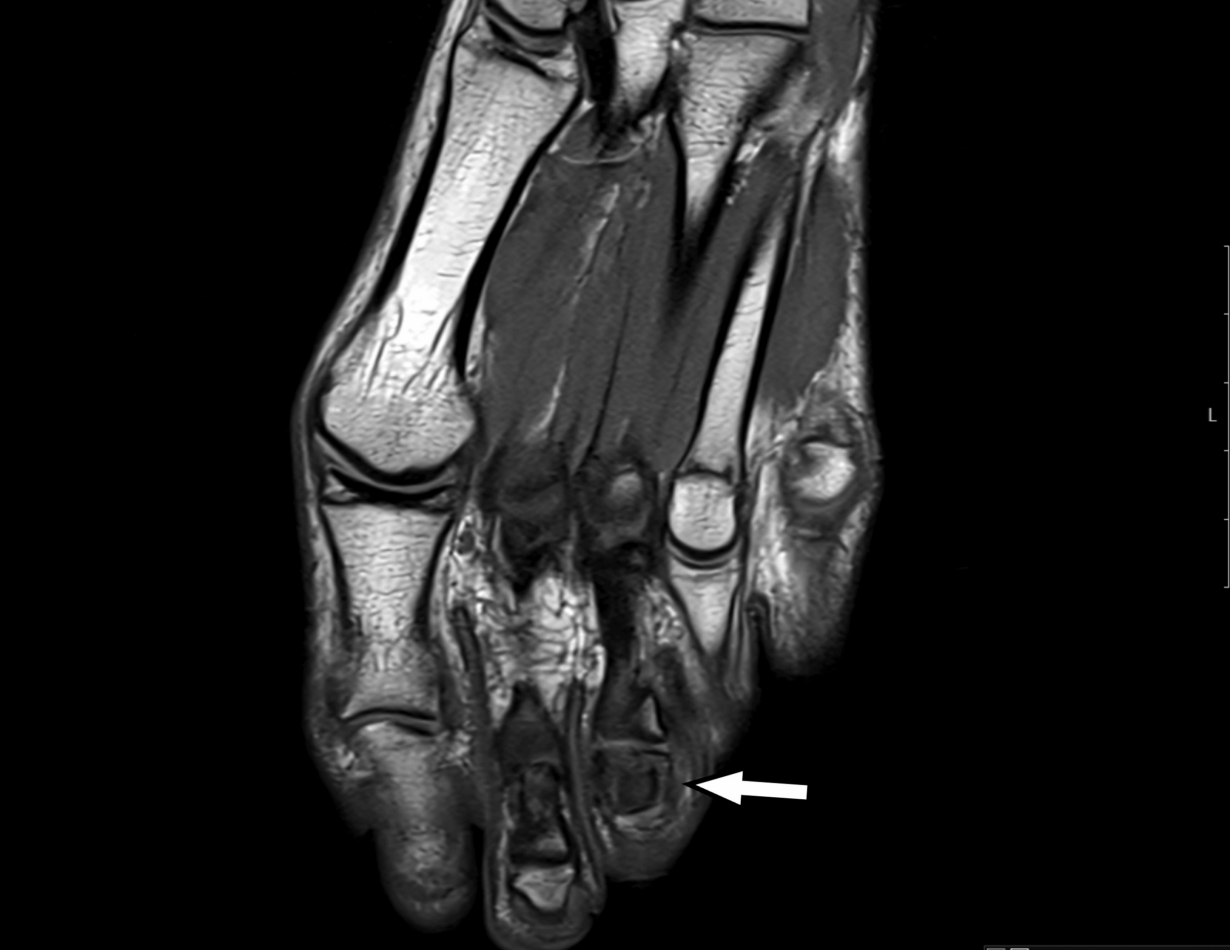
T1-weighted MRI showing an intermediate signal at the center of the lesion with low-signal intensity at the periphery
